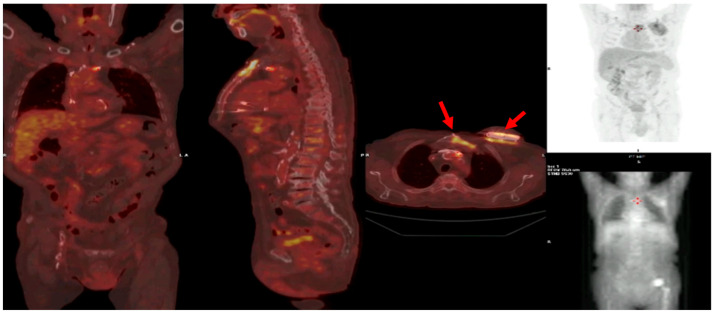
Patient with CIED endocarditis showing intense radiotracer uptake at the device pocket and catheter (red arrows) on this PET/CT scan. This is an original, de-identified clinical image from our institution. Patient consent for publication was obtained.
